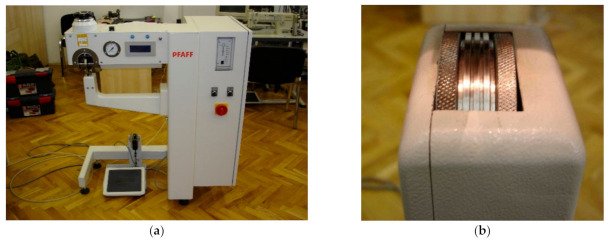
The machine for ultrasonic joining: (a) the machine; (b) lower engraving counter-roll.
